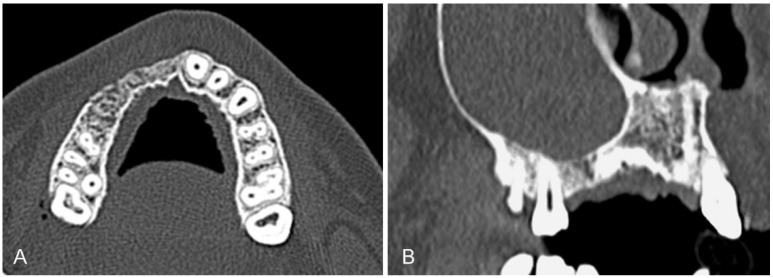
Dental CT-Scan at four months after trauma. Axial (A) and sagittal (B) CT-images (Images: Institute of Radiology, Kantonsspital Aarau, AG, Aarau, Switzerland).
